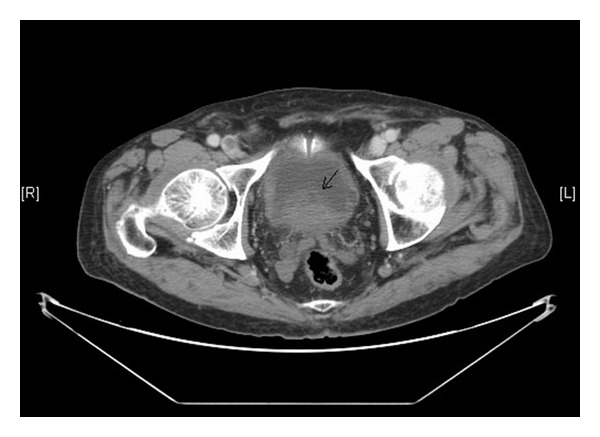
Computed tomography revealing a 5 cm size heterogeneous mass in the right wall and base of the bladder.
